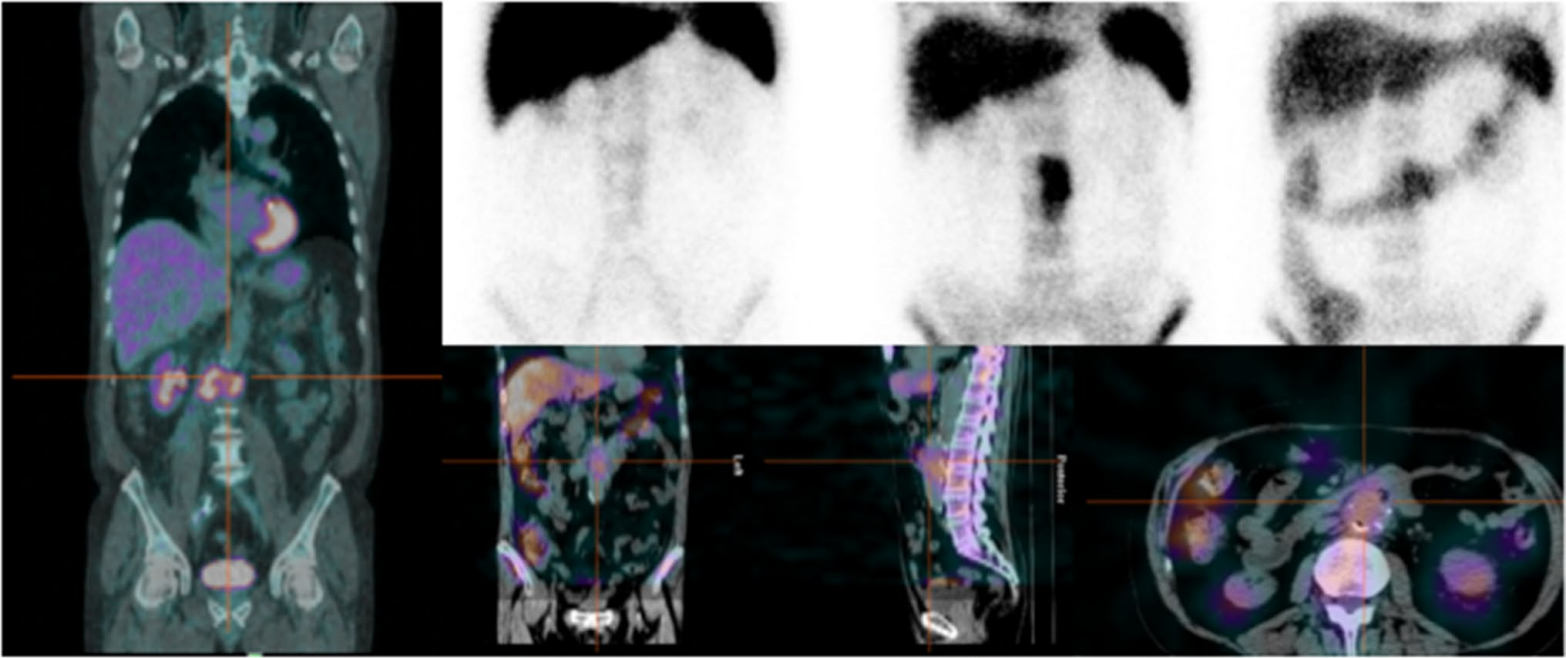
Example of concordantly positive PET/CT (left panel) and ^99m^Tc-WBC scintigraphy (planar images in upper right panel, 30’, 2 h and 20 h after injection; SPECT/CT images (in lower panel) in a patient with proved infection by Enterococcus faecium
